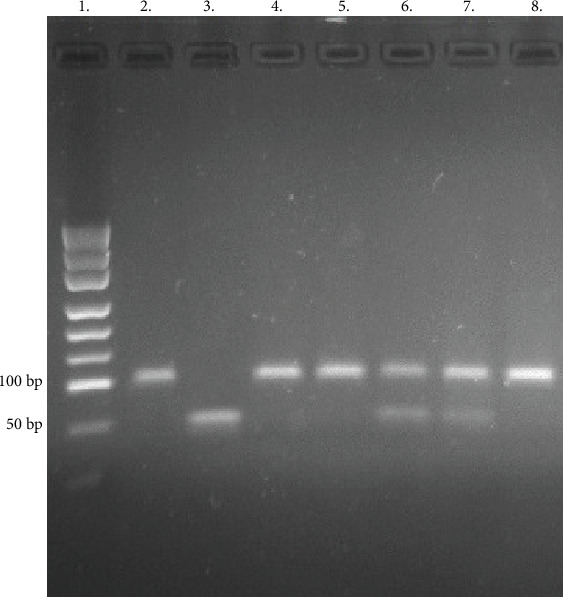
PCR-RFLP of *PSMA6* gene polymorphisms. Lane 1—ladder (34, 67, 110, 147, 190, 242 331, 404, and 504 bp). Lanes 2, 4, 5, and 8—CC genotypes. Lanes 6 and 7—CG heterozygotes. Lane 3—GG homozygote.
